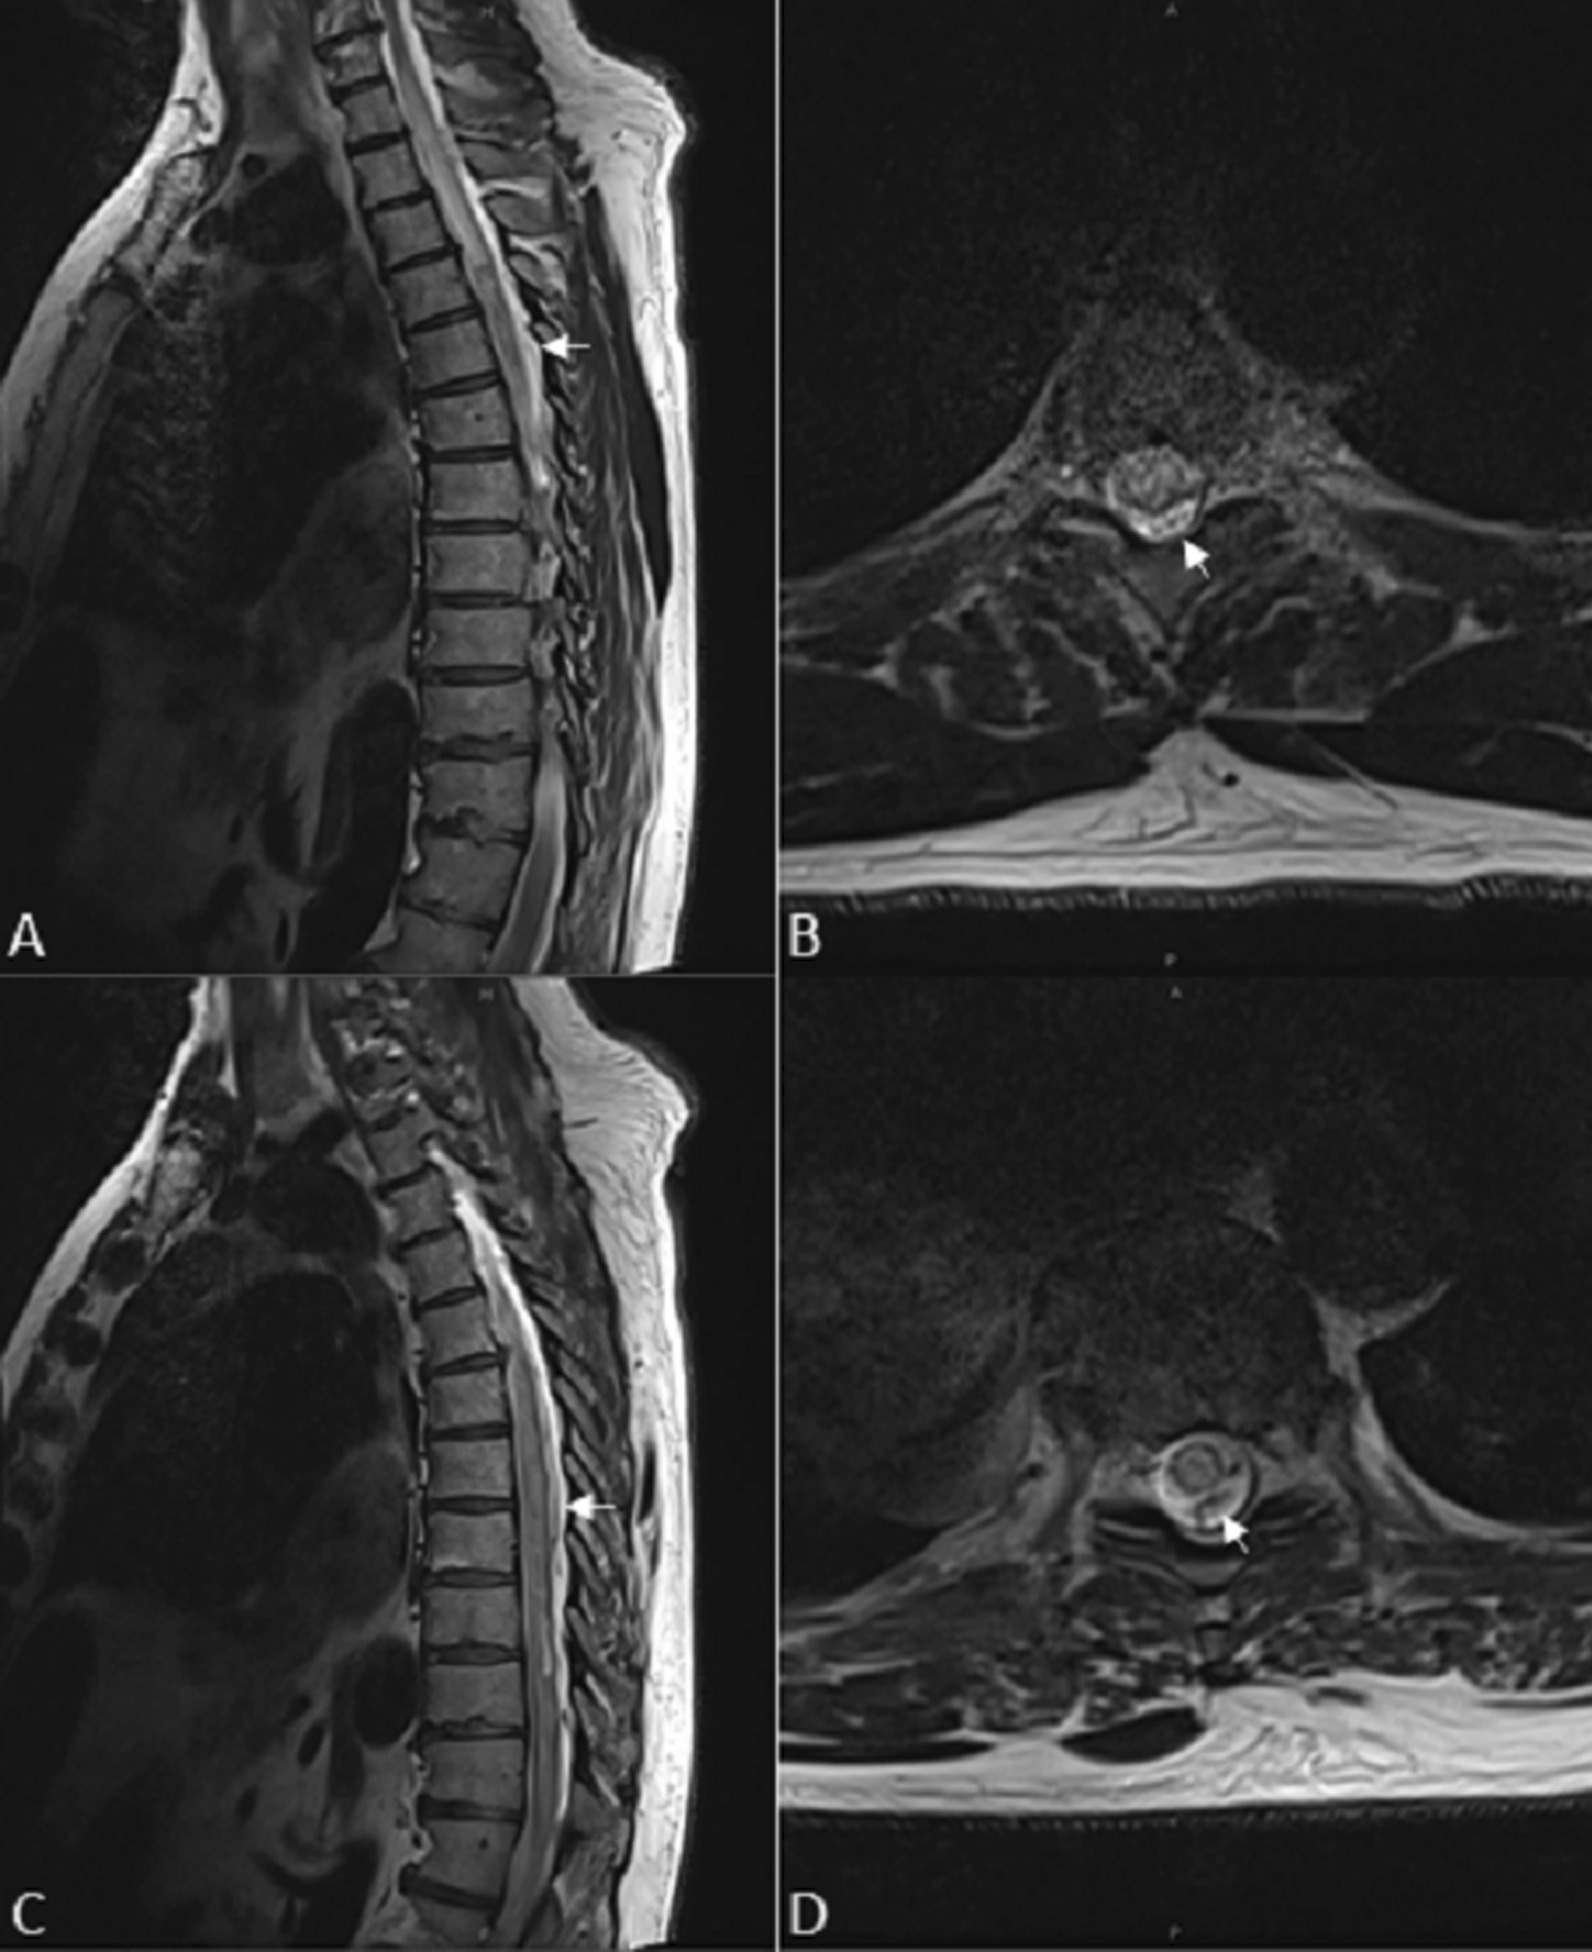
A Sagittal and (B) axial T2-weighted MR images of the cervicothoracic and (C, D) lower thoracic spine demonstrates diffuse cord expansion and heterogeneous hyperintensity from C7 to T8 with the severely dorsal-predominant serpiginous flow voids (arrows)
